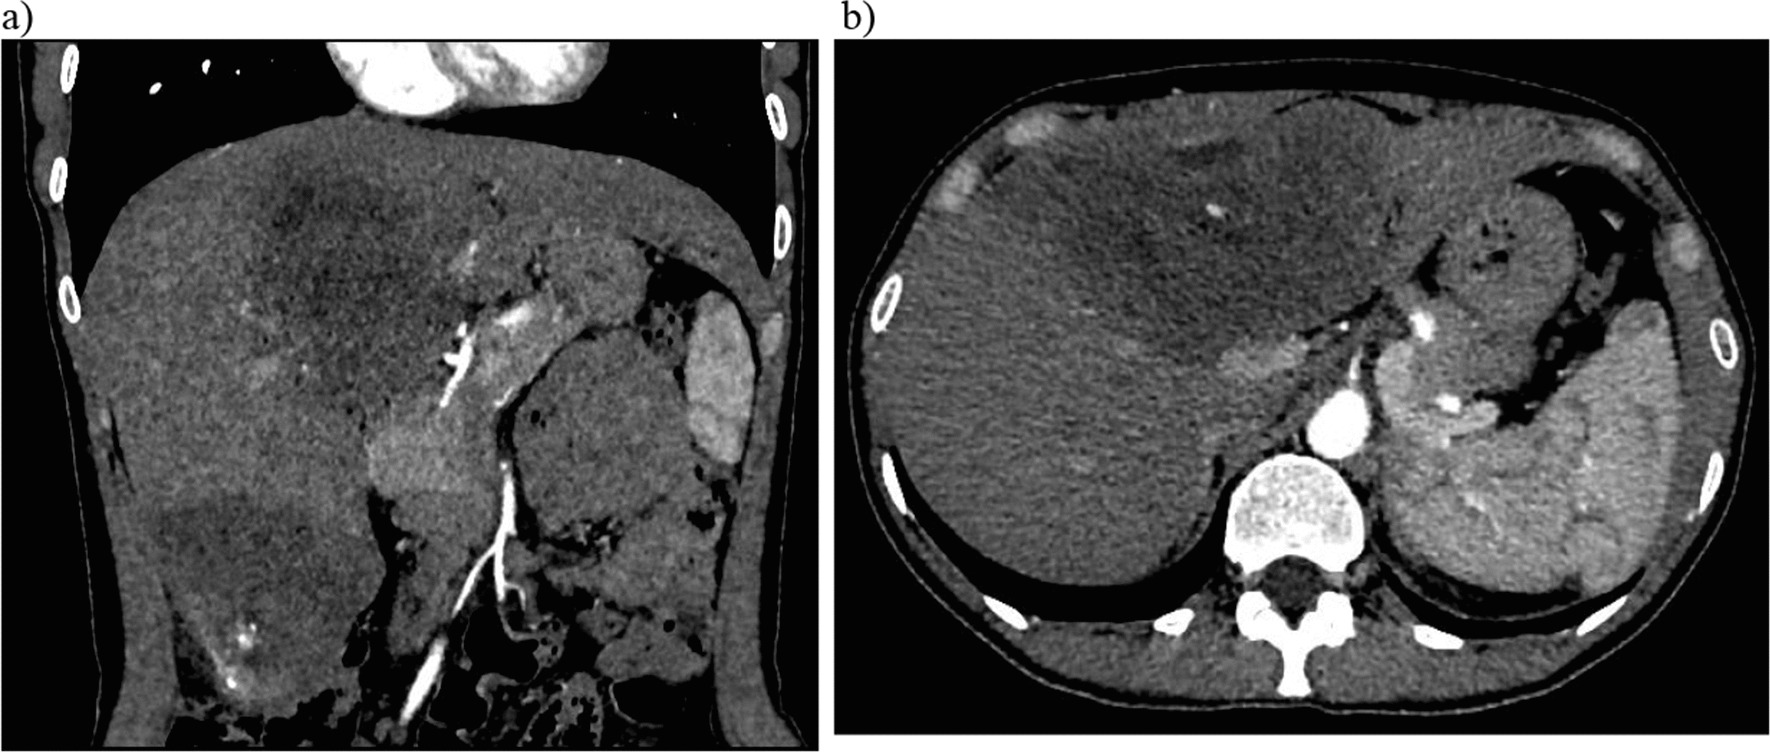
Contrast-enhanced coronal (a) and axial (b) CT images show in arterial phase there is a moderate accumulation of contrast agent in the solid part density up to + 45 to + 55 HU
